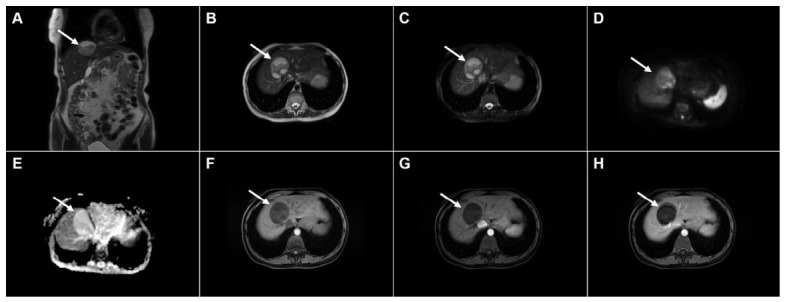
Images of a 77-year-old woman with cystic echinococcosis, WHO stage CE3b. Coronal T2-weighted image (A) demonstrates a heterogeneous cystic lesion (*arrow*) in liver segment VIII. Axial T2-weighted (B) and axial T2-weighted fat-suppressed (C) images show a few daughter cysts embedded within the hydatid matrix (*arrow*). The lesion (*arrow*) demonstrates no restricted diffusion in the diffusion-weighted image (D) and the corresponding ADC map (E). In the precontrast T1-weighted fat-suppressed image (F), the hydatid matrix exhibits a slightly higher signal intensity compared to the daughter cysts (*arrow*). No enhancement of the lesion (*arrow*) can be observed in arterial (G) or portovenous (H) phases.
